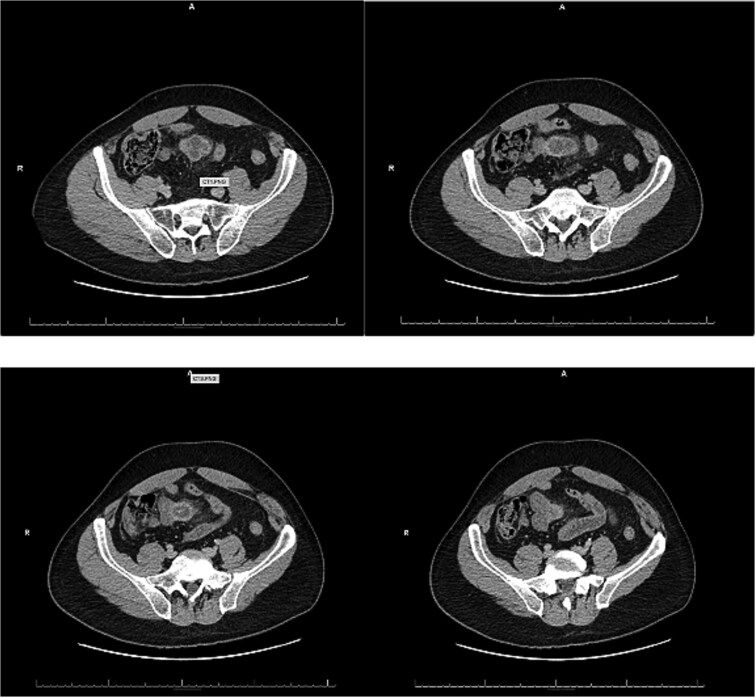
Representative slices of emergency department CT.
